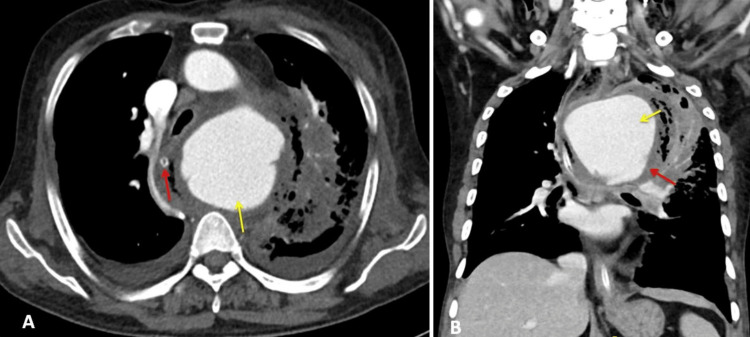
Computed tomography of the thorax. A (axial view): Aortic aneurysm (yellow arrow) and right side shift of the esophagus containing Ryle’s tube (red arrow). B (coronal view): Aortic aneurysm (yellow arrow) and eccentric thrombus (red arrow)
